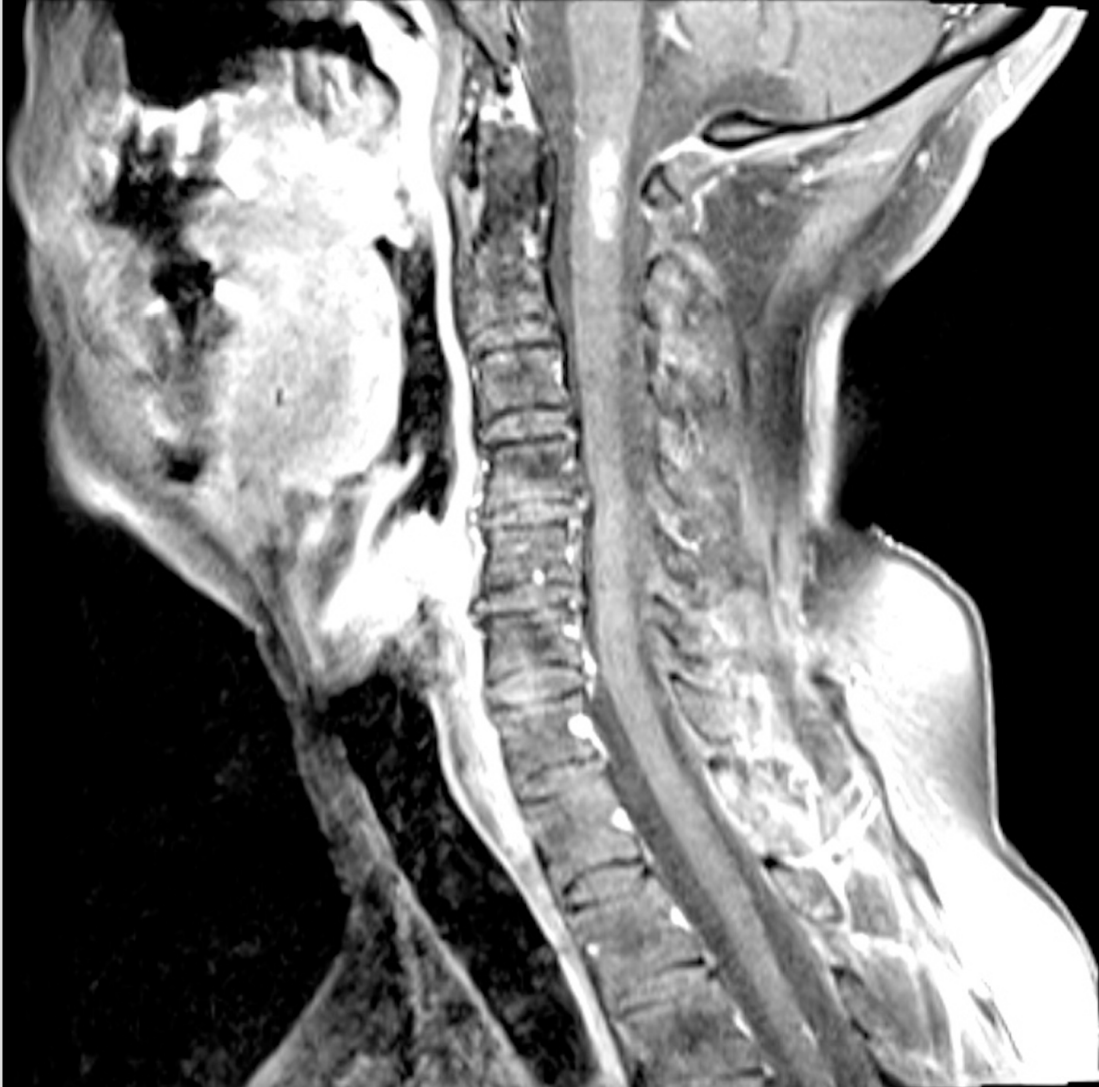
Cervical Spine MRI showing rapid enlargement Sagittal MRI of the cervical spine demonstrated substantial and rapid enlargement of the contrast enhancing lesion at the cervicomedullary junction.
